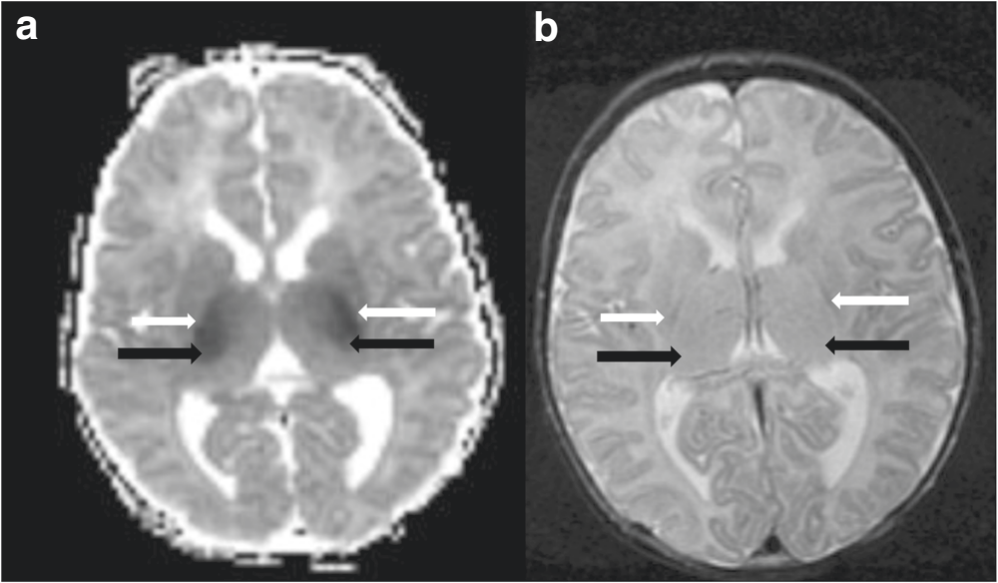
Acute, deep gray pattern of hypoxic ischemic brain injury. a ADC map demonstrating restricted diffusion involving the posterior limbs of the internal capsules (white arrows) and ventrolateral thalami (black arrows) and b T2-weighted imaging demonstrating hyperintensity involving the posterior limb of the internal capsule (white arrows) and bilateral thalami (black arrows).
